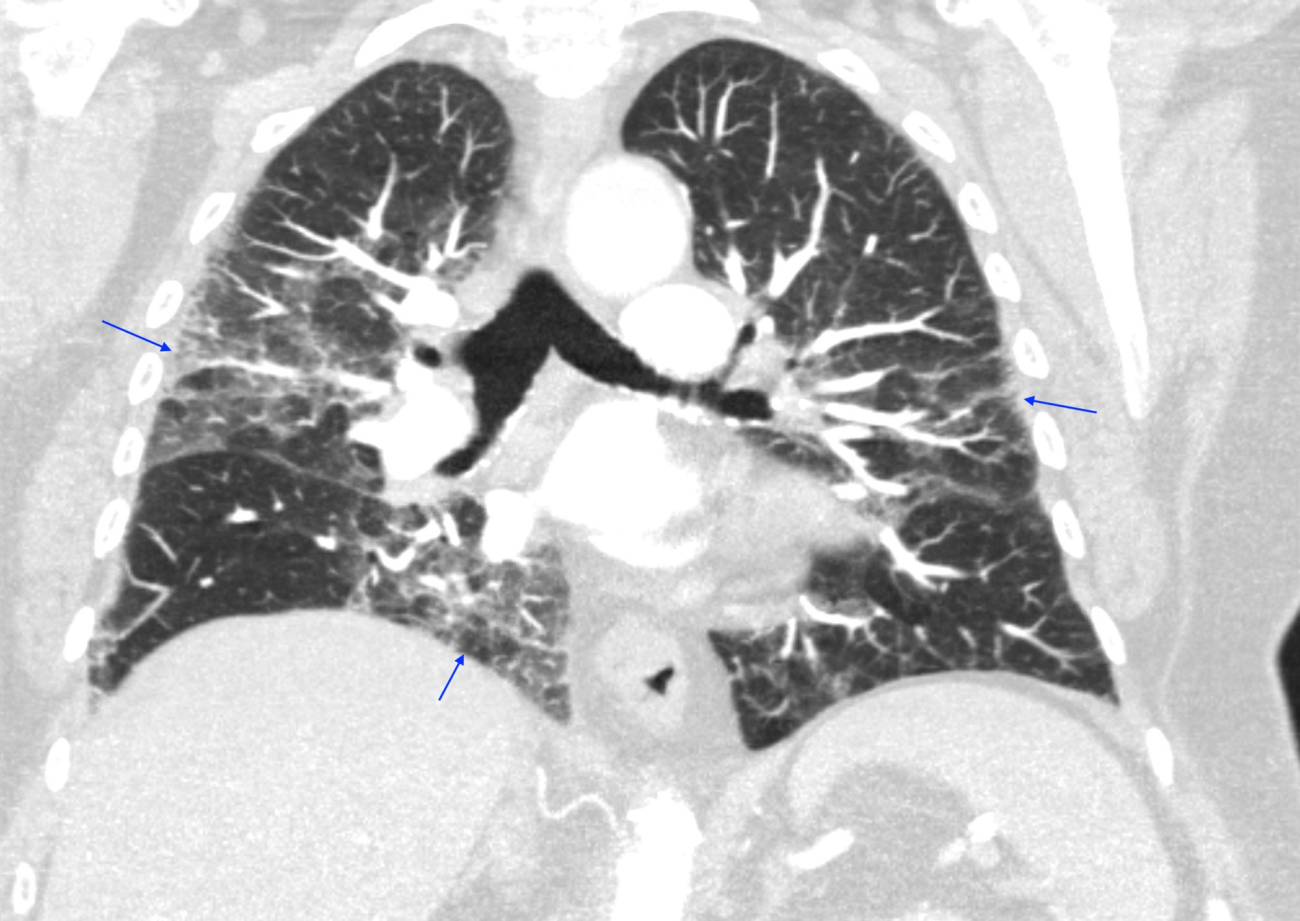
CT Chest (Coronal View) Bilateral patchy ground-glass opacities without intraluminal defects, cardiomegaly, or pleural effusions.
